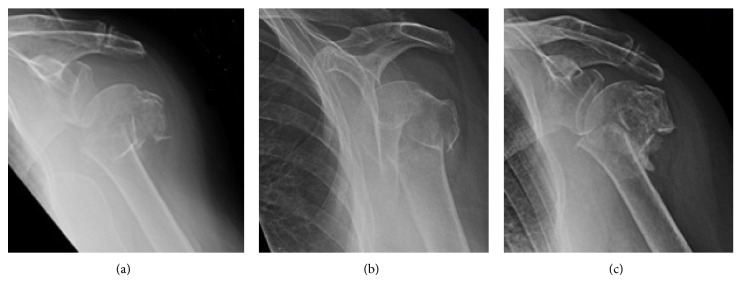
AP radiographs of left proximal humerus showing initial displacement (a) and reduction with conservative management (b), leading to painful nonunion at 4-month stage.
